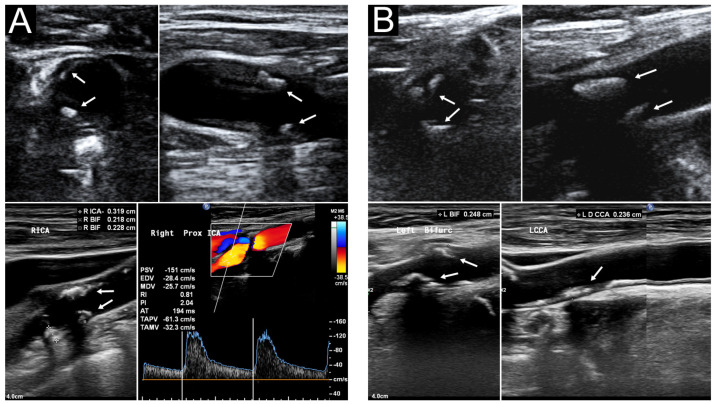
Carotid ultrasound of a 91−year−old woman. Her outpatient sCPS, laboratory sCPS, and laboratory hCPS were 6, 6, and 19.5, respectively. (A) Segmental heteroechogenic plaques (arrows) were detected in the right carotid bifurcation (BIF) and internal carotid artery (ICA) through transverse (upper left) and longitudinal (upper right) POCUS performed in the outpatient department. Laboratory sonography revealed the same segmental plaques more clearly with various plaque thickness (lower left). Color duplex sonography indicated an elevated peak systolic velocity in the ICA (lower right). (B) Segmental plaques (arrows) were detected in the left carotid BIF and ICA through transverse (upper left) and longitudinal (upper right) approach POCUS. Laboratory sonography indicated more segmental plaques extending to the common carotid artery (lower right). BIF, right carotid bifurcation; hCPS, Handa’s carotid plaque score; ICA, internal carotid artery; POCUS, point-of-care ultrasound; sCPS, simplified carotid plaque score.
